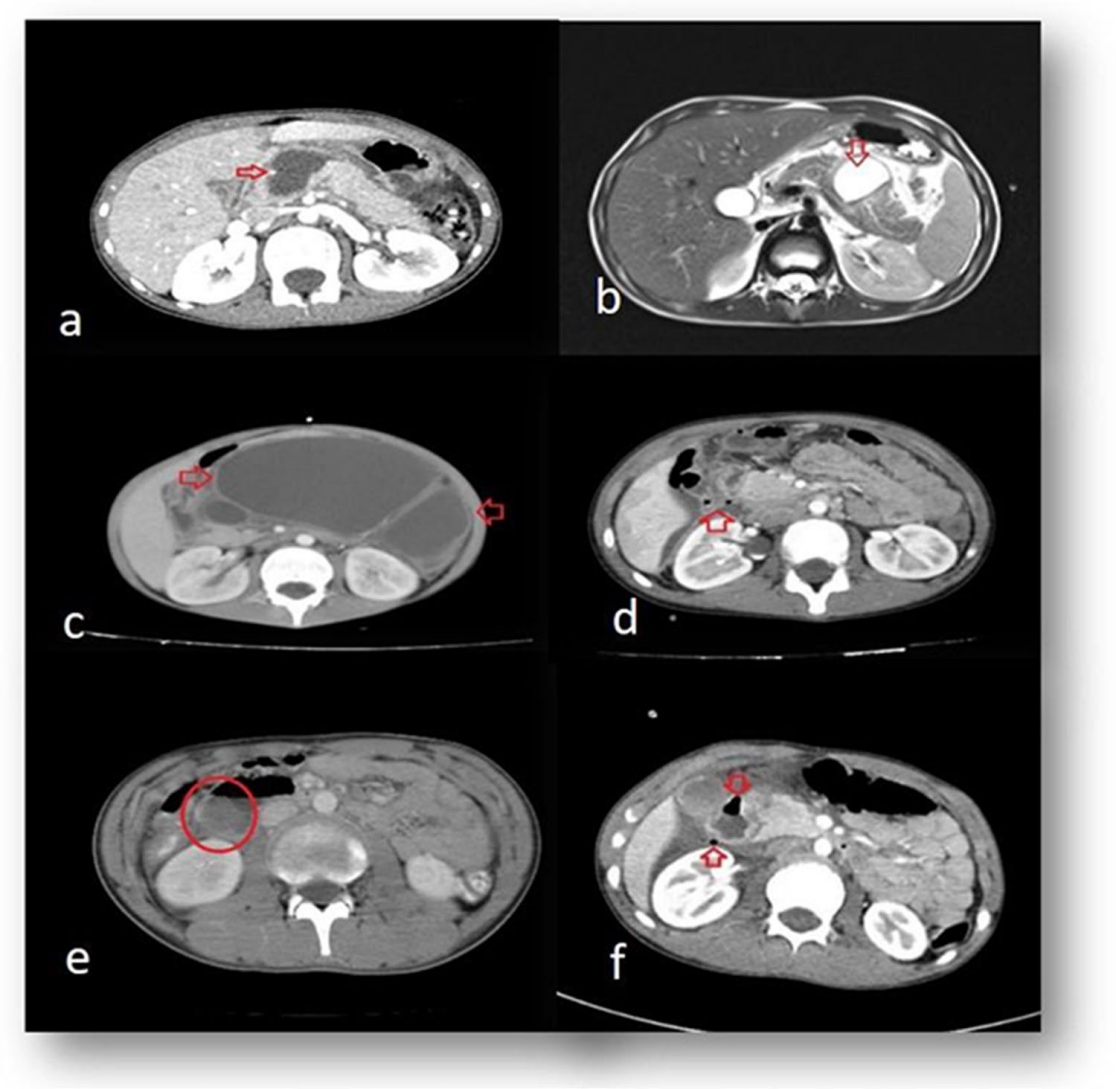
Various CT and MRI images of the pancreatic/duodenal trauma cases. a Pancreatic laceration on abdominal CT (arrow); b pancreatic laceration on abdominal MRI (arrow); c pseudocyst image on abdominal CT (arrows); d image of free air belonging to duodenal perforation on abdominal CT (arrow); e duodenal hematoma on abdominal CT (circle); f impaired duodenal wall integrity and free air on abdominal CT (arrows)
